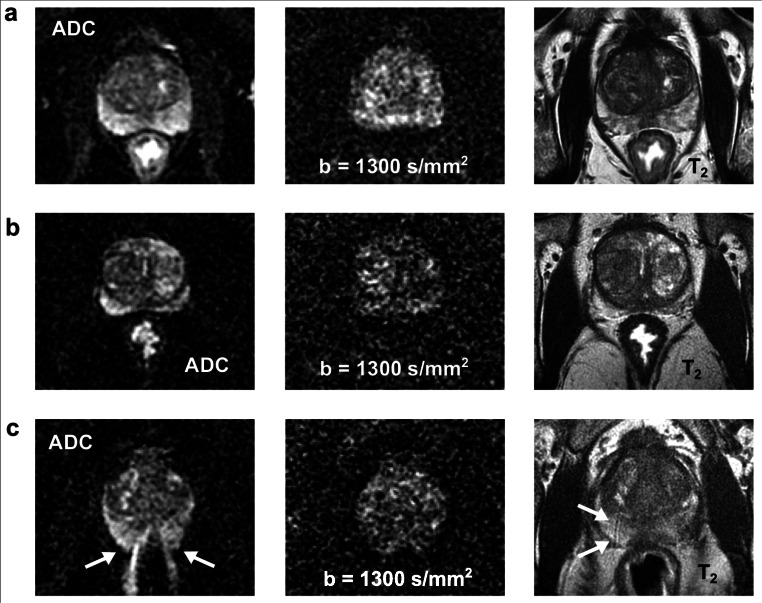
Exemplary ADC maps as well as diffusion-weighted and T_2_-weighted images of three patients with enema preparation: a A 77-year-old patient with a collapsed rectum and excellent DWI quality with respect to the severity of susceptibility-induced artifacts, the differentiability of the anatomy, and the overall image quality (both readers). b A 65-year-old patient with a small amount of liquid/fluid in the rectum but still excellent DWI quality (both readers). c A 59-year-old patient with a moderate amount of air in the rectum which led to severe image distortions (see arrows), poorly identifiable anatomy, and poor overall image quality; motion and blurring artifacts are visible on the T_2_-weighted image (see arrows, both readers)
